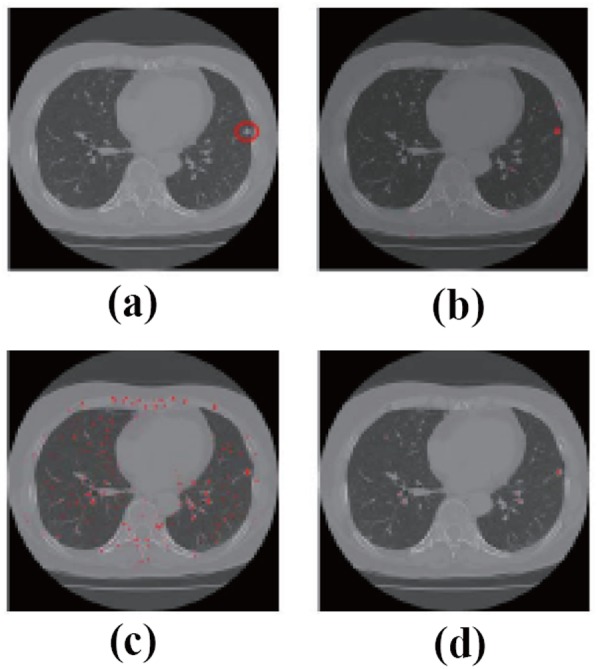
a, Original CT Image; b, Enhanced nodules by 3D multi-scale filter; c, 2D Multi-scale filter; d, Takemura’s proposed method
